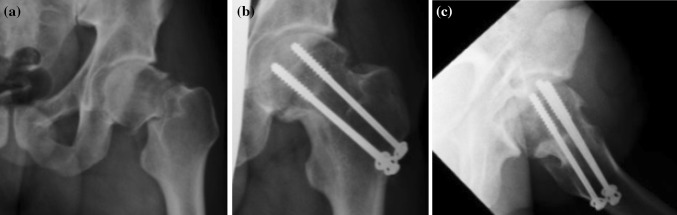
Intracapsular femoral fracture a anteroposterior X-ray, b Osteosynthesis with three lag screws (anteroposterior X-ray), c Osteosynthesis with three lag screws (lateral X-ray)
